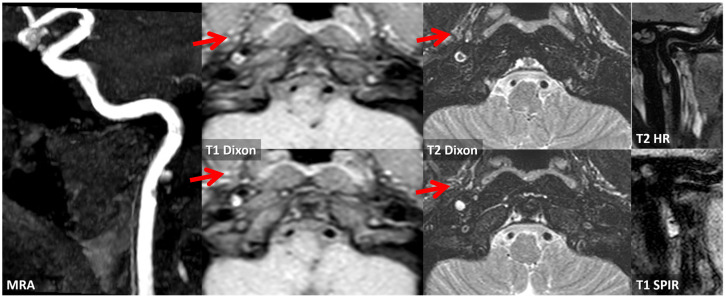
MRI study at 20 days from the first neuroimaging investigation. TOF MRA with MIP/MPR reconstruction in oblique plane confirmed the presence of an ulcerated atheroma on the prepetrous segment of the right ICA (red arrows). Both T1 and T2 Dixon axial sequences at the level of the proximal part of the atheroma and at the level of the ulceration show a hyperintense core and a linear peripheral hyperintensity. T1 HR and T2 SPIR sequences in oblique plane show the same signal features of a complicated atheroma along its longitudinal extension with positive remodeling of the involved ICA segment.
